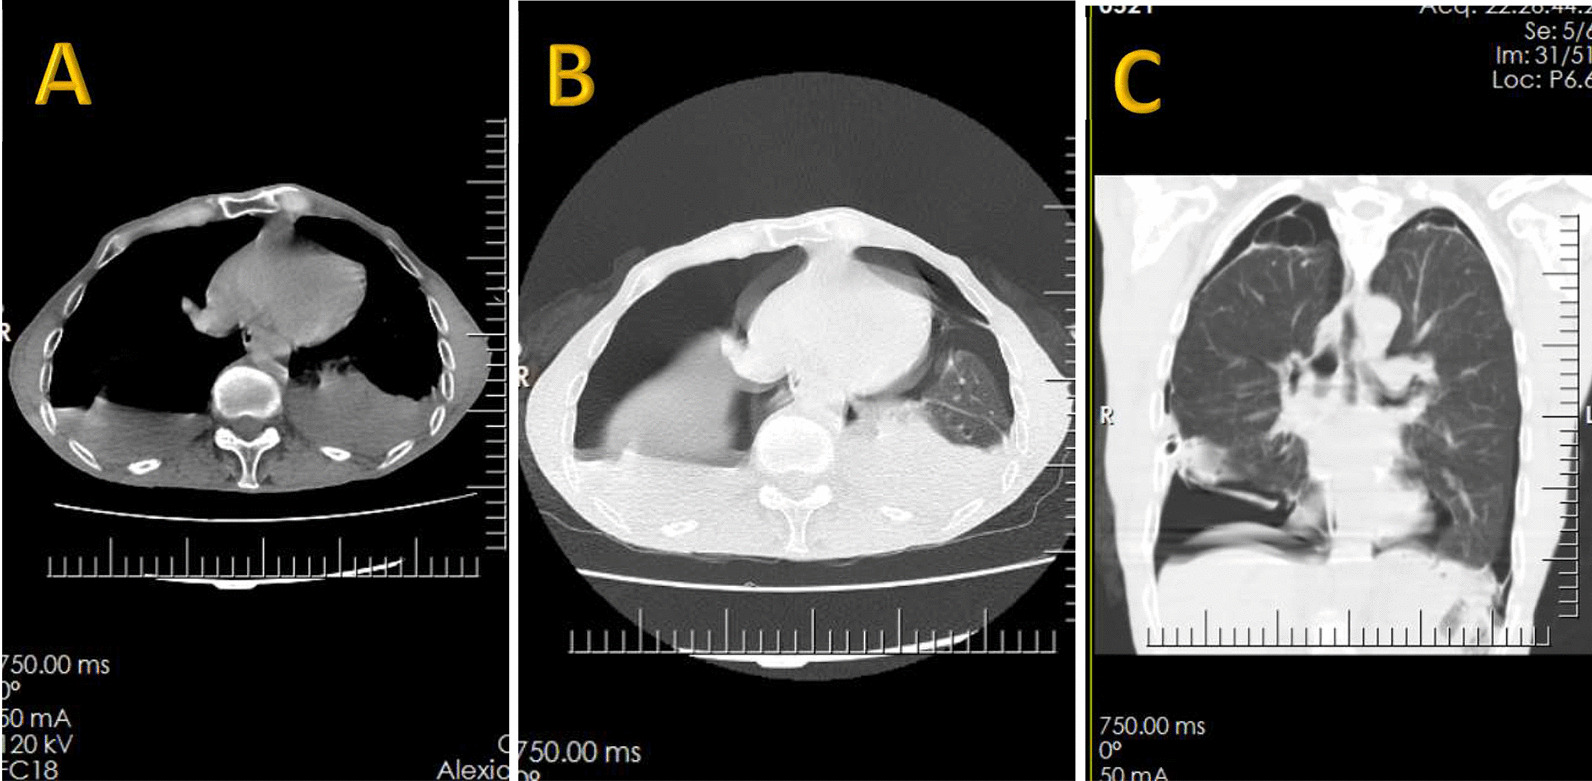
Spiral chest CT scan without contrast (1st admission), bilateral primary spontaneous pneumothorax, a mediastinal axial view, b lung window axial view, c lung window coronal view
